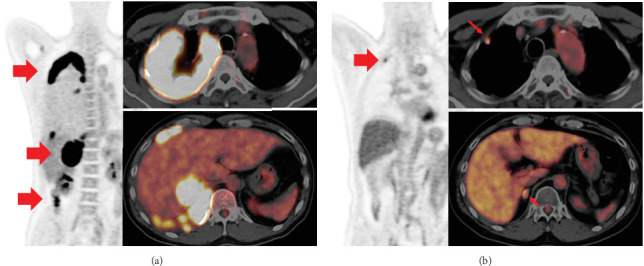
PET-CT scan (a) before ICI therapy and (b) after four treatment cycles. The patient has achieved partial response (PR). ICI, immune checkpoint inhibitor.
